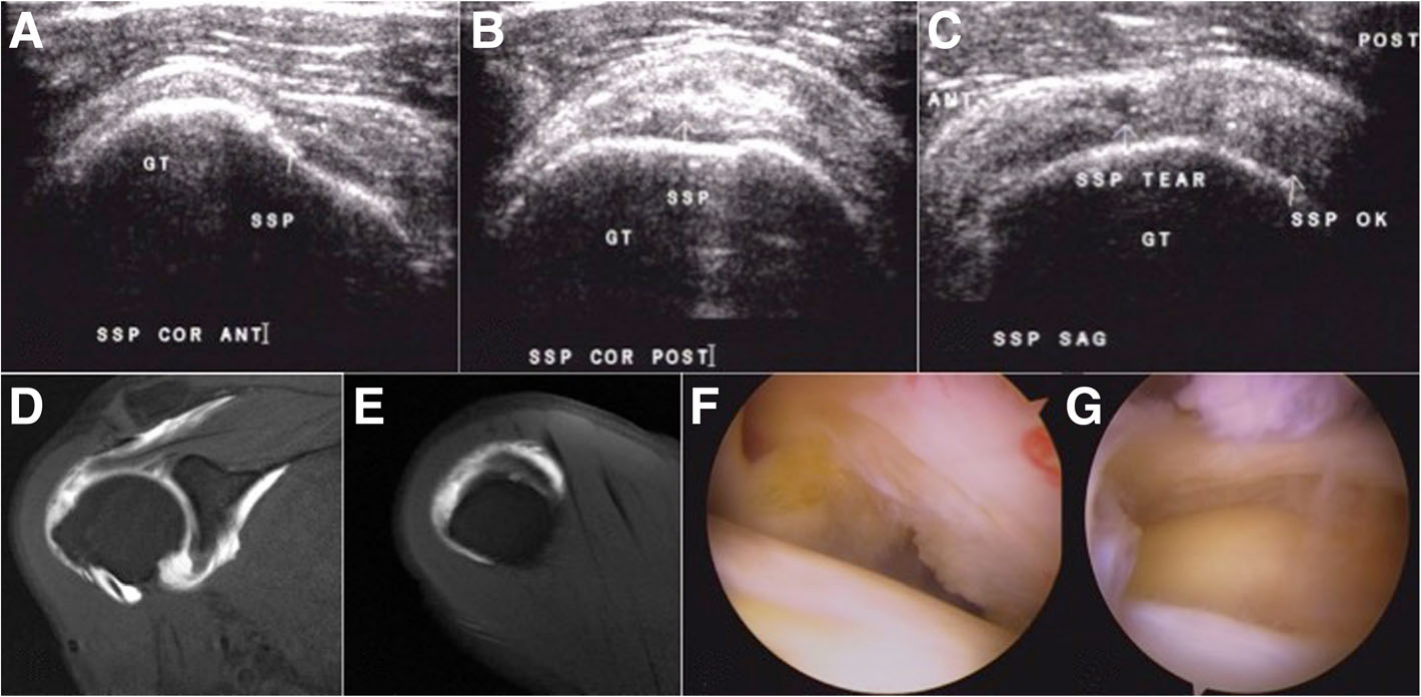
Case 1. A 60-year-old male patient with a full-thickness tear of the anterior part of the supraspinatus tendon. a Coronal ultrasonographic view of the anterior part of the supraspinatus. b Coronal ultrasonographic view of the posterior part of the supraspinatus. c Sagittal ultrasonographic view of the lateral part of the supraspinatus. d Coronal MRI view of the anterior part of the supraspinatus. e Sagittal view of MRI of the lateral part of the supraspinatus. f Intra-articular view of the anterior part of the supraspinatus tendon tear. g Subacromial view of the anterior part of the supraspinatus tendon tear
